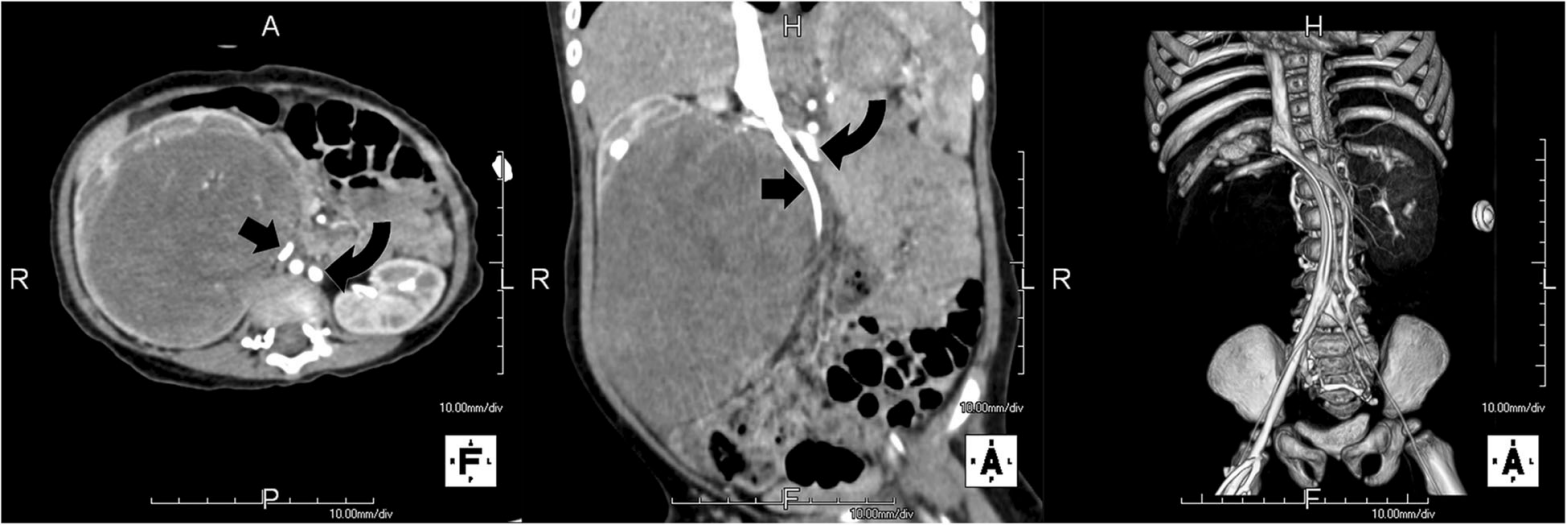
Axial, coronal and three-dimensional reconstruction computed tomography scan with contrast showing a large mass in the right kidney, duplication of the inferior vena cava below the renal veins and compression and displacement of the right inferior vena cava. Straight arrow denotes the right inferior vena cava, and curved arrow the left inferior vena cava
